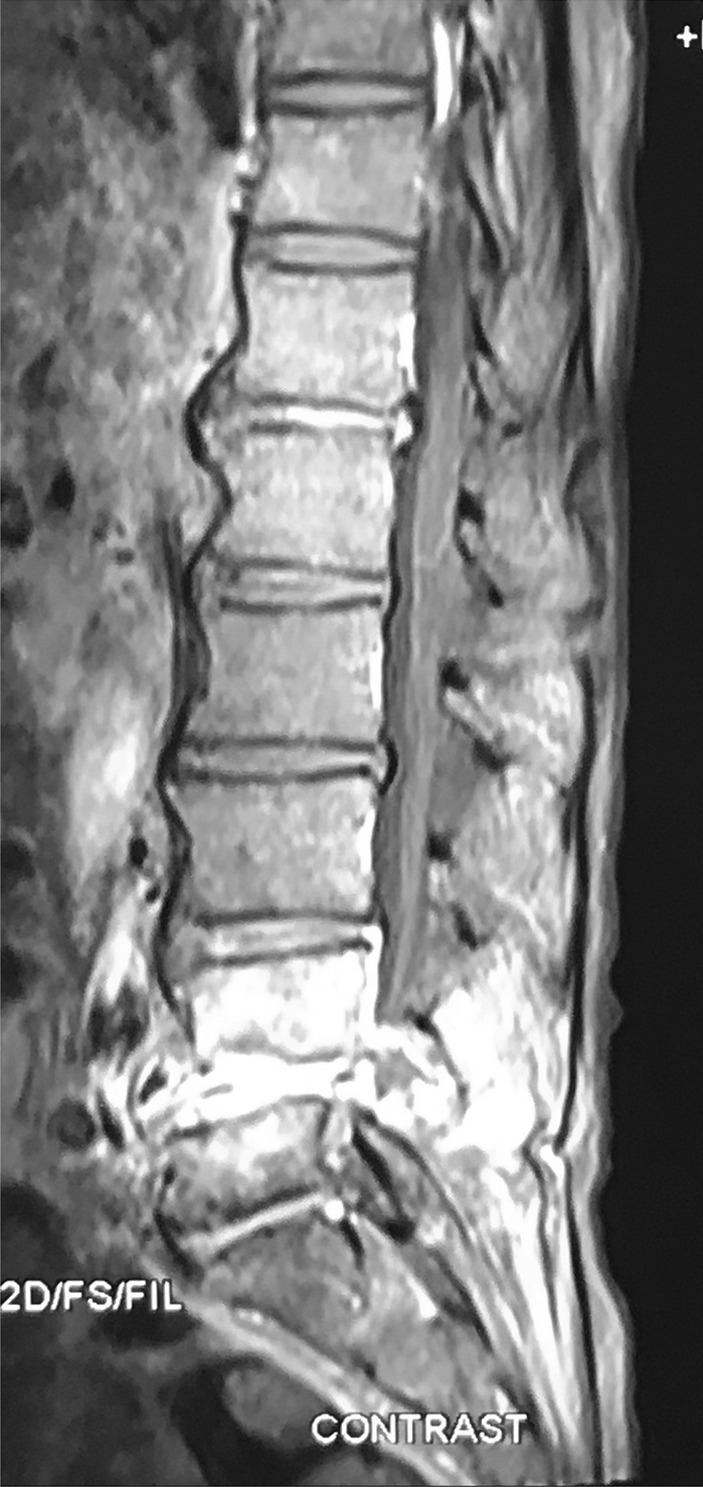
MRI lumbosacral spine sagittal contrast T1-weighted image shows avid contrast enhancement
